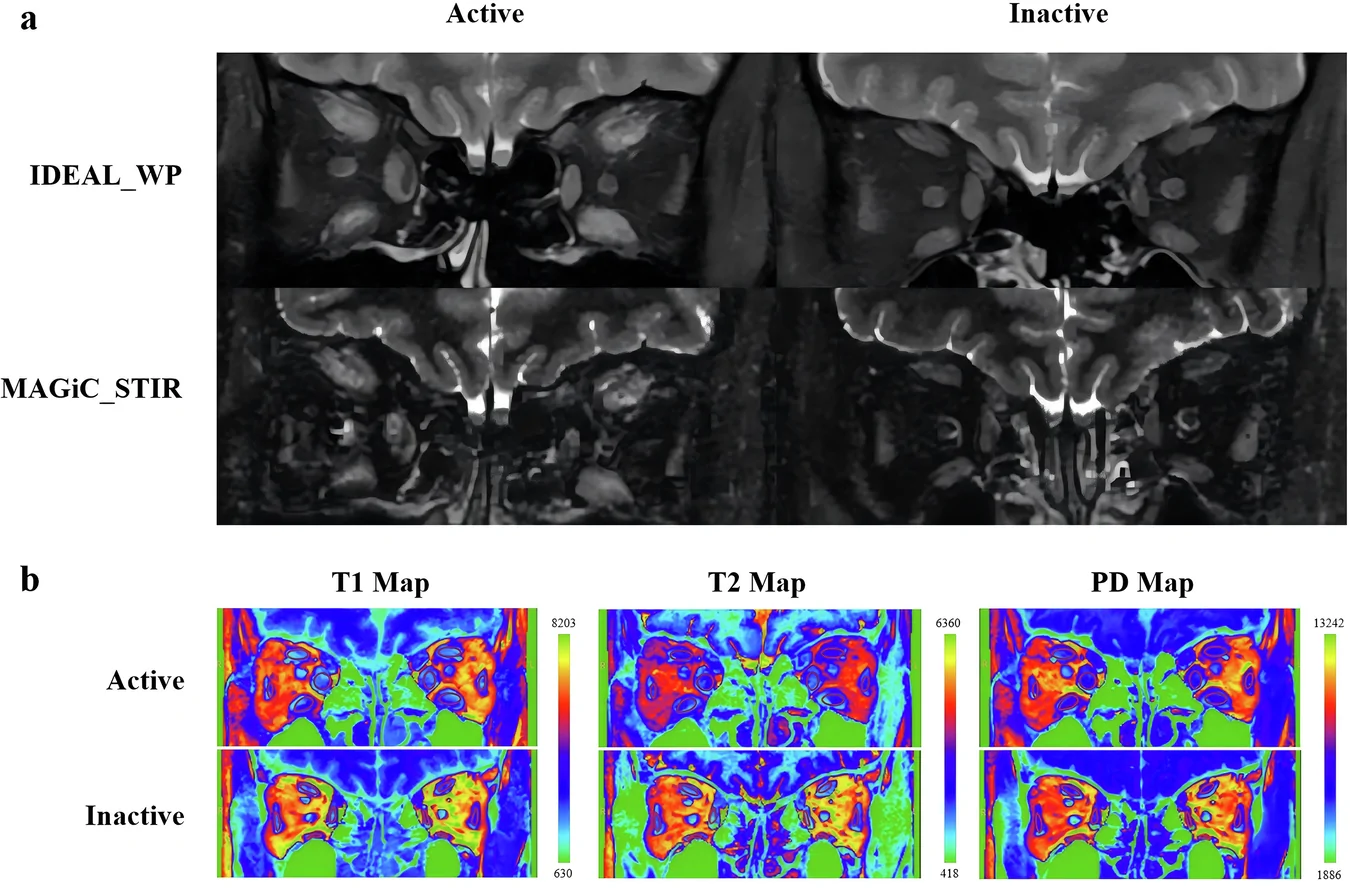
Comparison of IDEAL IQ_WP and MAGiC_STIR images and quantitative maps between active and inactive TED. a The morphologic structures observed in both IDEAL IQ_WP and MAGiC_STIR. b Representative images for T1 map, T2 map, and PD map, respectively, of an active and inactive TED subject. IDEAL IQ, Iterative decomposition of water and fat with echo asymmetry and least-squares estimation quantitation; MAGiC, Magnetic resonance image compilation; PD, Proton density; STIR, Short tau inversion-recovery; WP, Water phase
